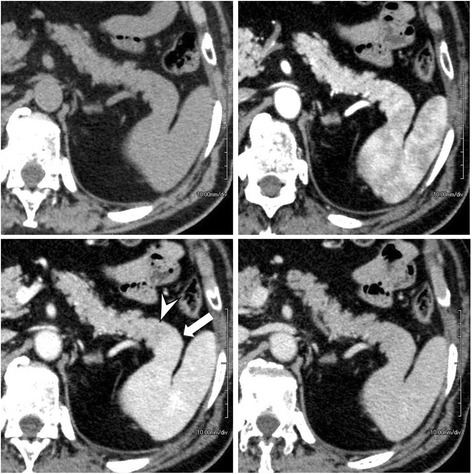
Axial triple-phase contrast-enhanced multidetector-row computed tomography images show the pancreatic tail in continuity with the spleen. The boundary between the organs is difficult to identify on unenhanced multidetector-row computed tomography (*upper left*); however, in the portal venous phase (*lower left*), the boundary between the pancreas (*arrowhead*) and spleen (*arrow*) is clearly visible
